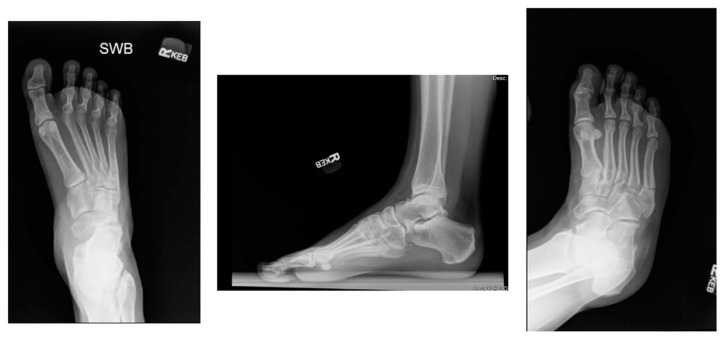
Weight-bearing AP, lateral, and oblique radiographs of an acute, non-displaced Jones fracture before ORIF in a 21-year-old male.
